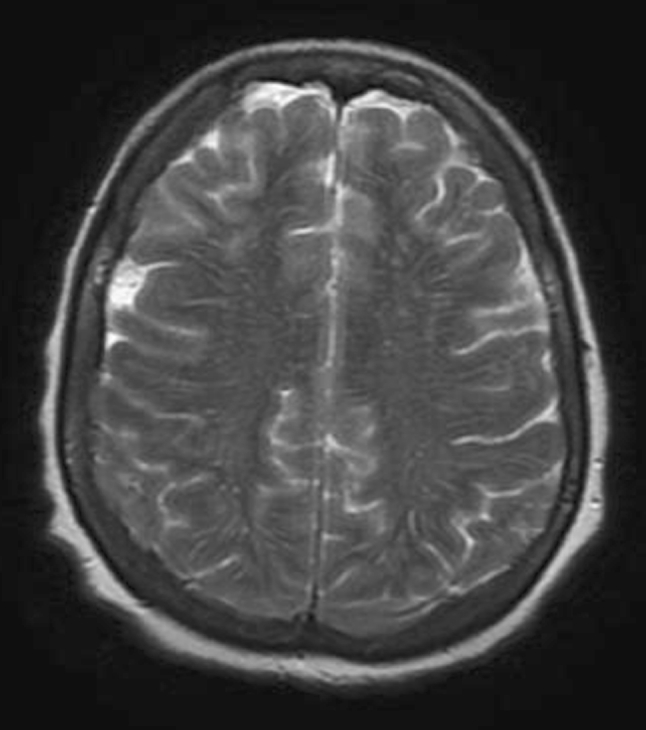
MRI (GE-Philips 1.5T) Patient’s axial T2 weighted image (*top of the head*: absence of meningeal abnormalities)
